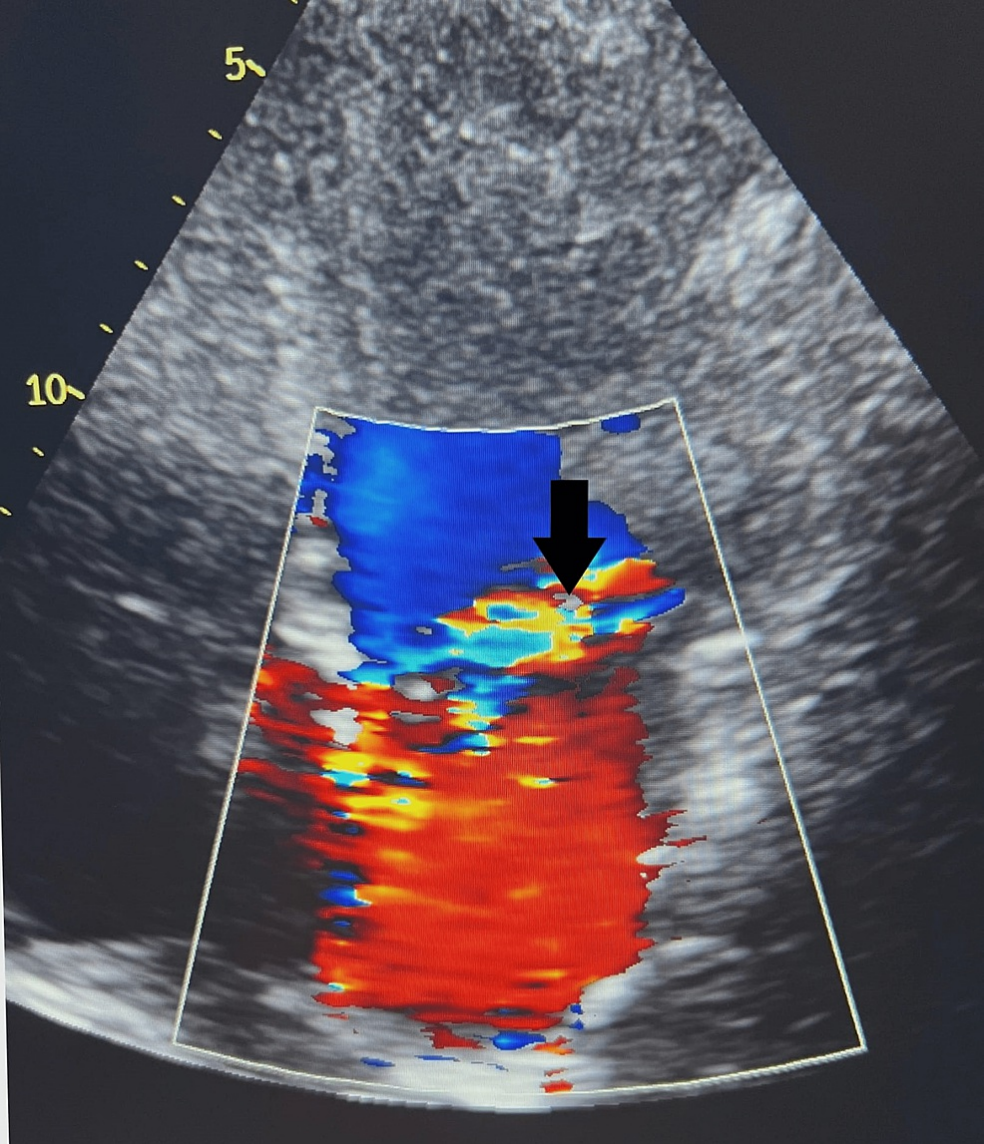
Echocardiography Arrow indicates severe mitral regurgitation
